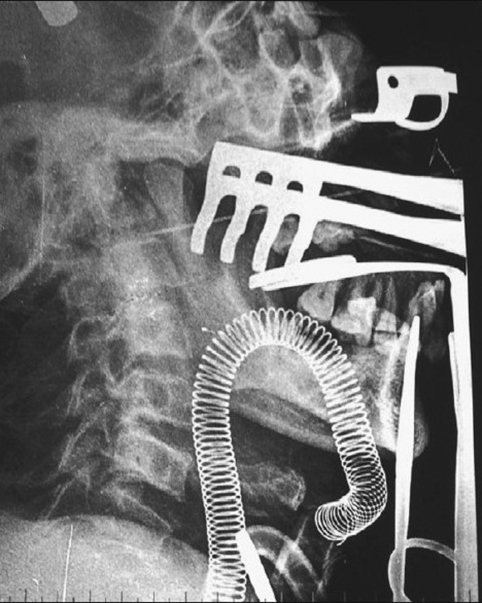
Intraoperative radiograph showing course of the submental intubation
